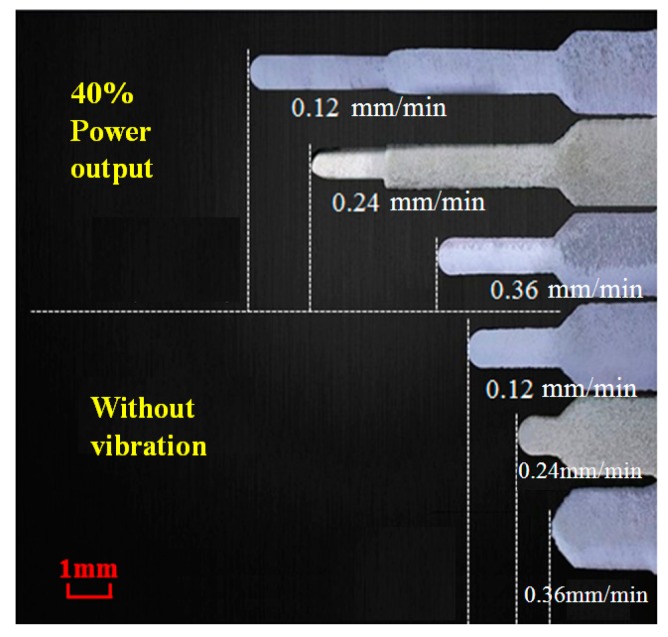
Ultrasonic extrusion length of Zr35 MG at various microextrusion rates.
